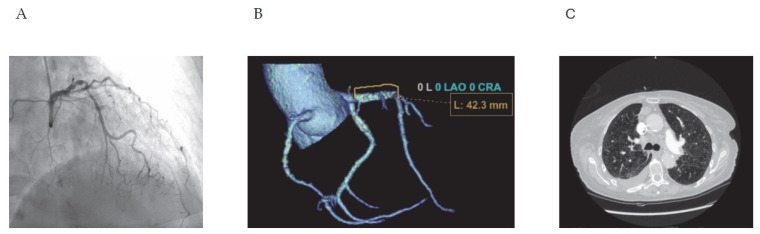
(A) Coronary angiography. (B) Coronary CT angiography. (C) Chest CT.
